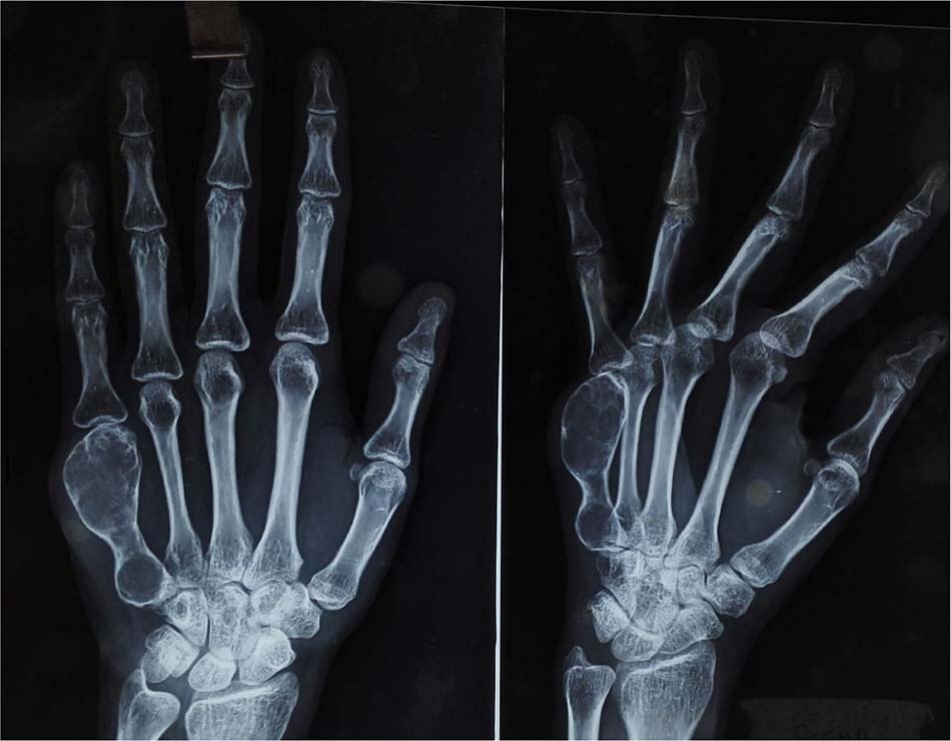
X-ray of the right hand shows an expansile, lytic lesion in the diaphysis of the fifth metacarpal with a “paper-thin” cortex extending to the subchondral bone.
